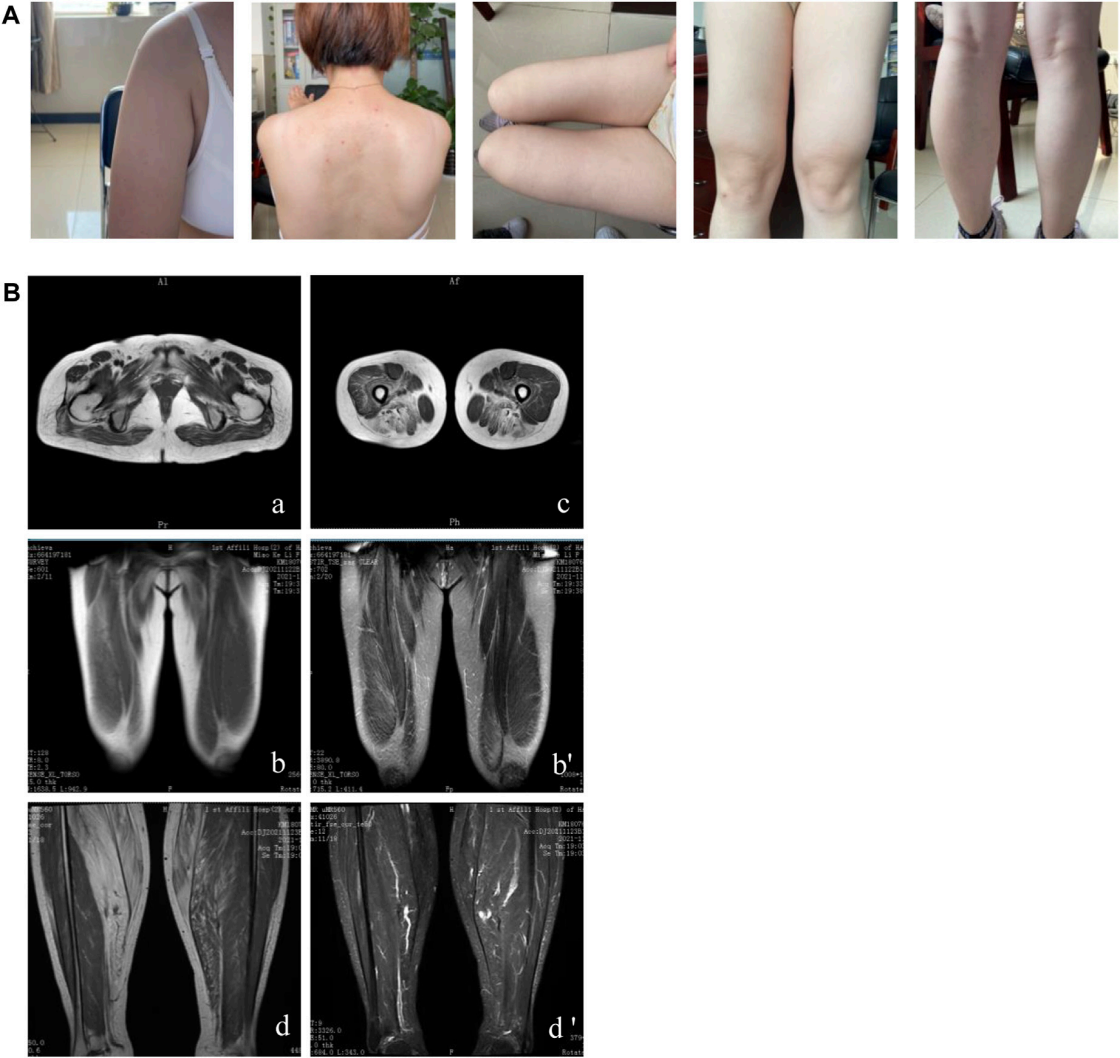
Clinical features and muscular MRI of patient II-3. (A) Winged scapulae, atrophy of the deltoid, inner muscles of the thigh and right calf, and left calf pseudo-hypertrophy are shown. (B) The affected glutei/iliopsoas muscles (A), vastus lateralis/gracilis (B), adductors/biceps femoris/semitendinosus and semimembranosus muscles (C), and gastrocnemius/soleus (D) were presented through muscular MRI.
